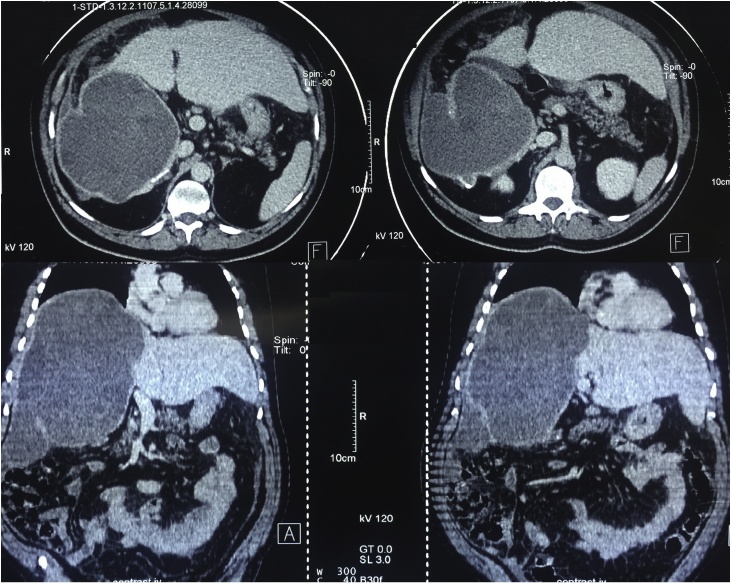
CT-scan of the abdomen showing a large hydatid cyst in the right lobe of the liver with fluid collection around the cyst.
